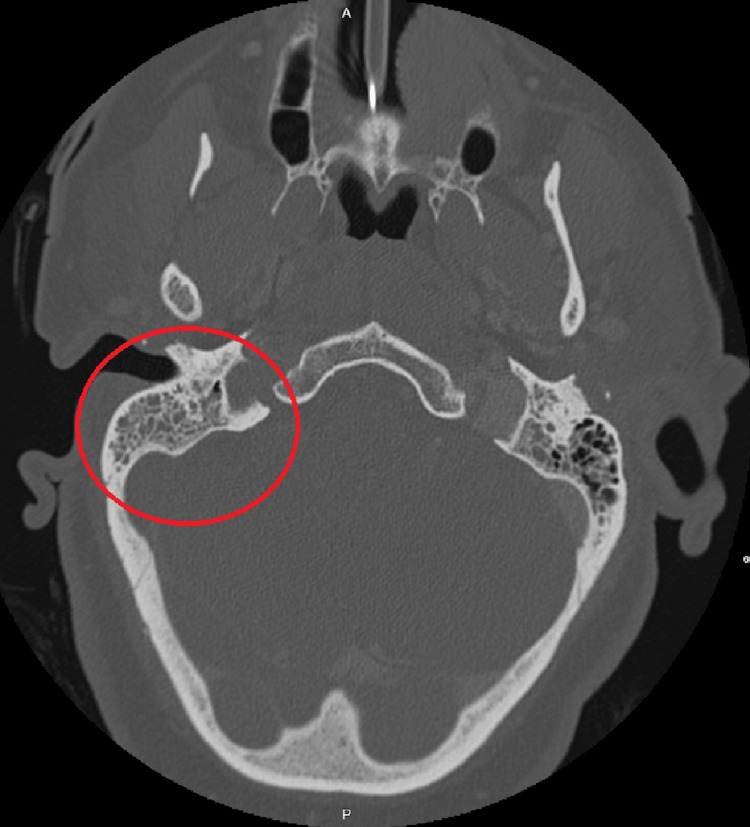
CT scan of the orbit and sella with contrast Opacification of the mastoid cells is present on the right side and is further highlighted by the red circle. The left mastoid bone is unremarkable without demonstrable middle ear disease or bone destruction. CT: computed tomography
